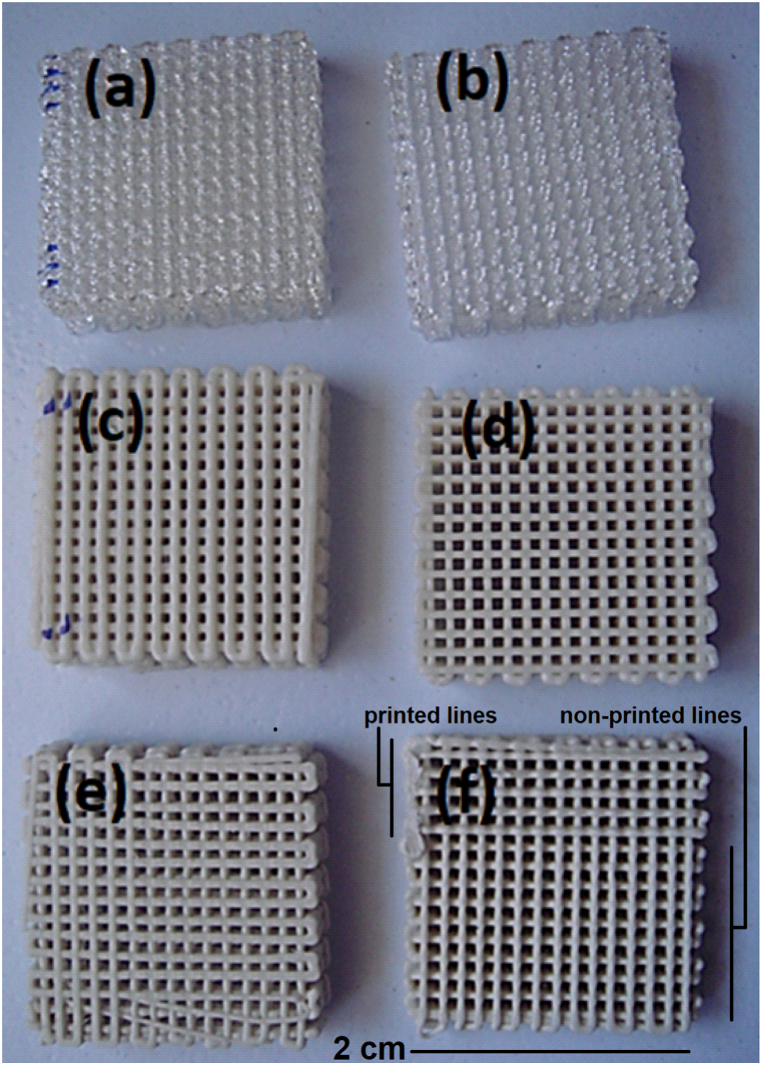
Top view of the printed scaffolds. (a and b): PLA scaffolds fabricated by ultrasonic-assisted 3D printing and conventional 3D printing; (c and d): PLA-20Ak scaffolds fabricated by ultrasonic-assisted 3D printing and conventional 3D printing; (e and f): PLA-40Ak scaffolds fabricated by ultrasonic-assisted 3D printing and conventional 3D printing. The samples have dimensions of 20 × 20 × 5 mm.
